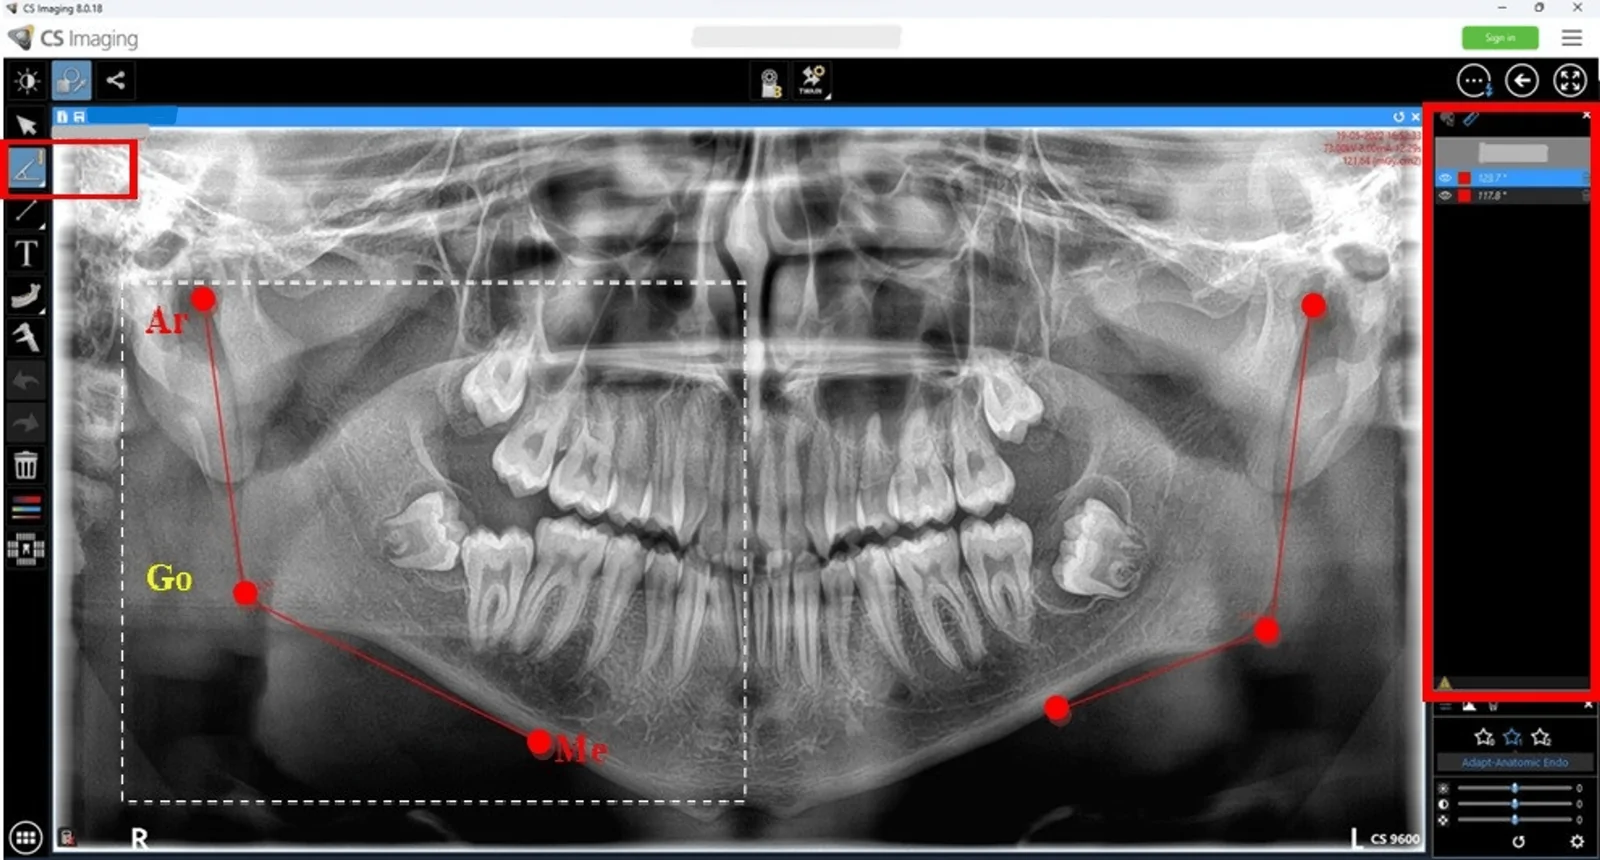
Orthopantomogram showing the gonial angle (Ar-Go-Me; red), measured between a line tangent to the posterior border of the mandibular ramus and a line tangent to the inferior border of the mandible. The measurement was obtained using the angle measurement tool in Carestream 9600 imaging software, version 8.018. Ar: Articulare; Go: Gonion; Me: Menton; OPG: Orthopantomogram.
